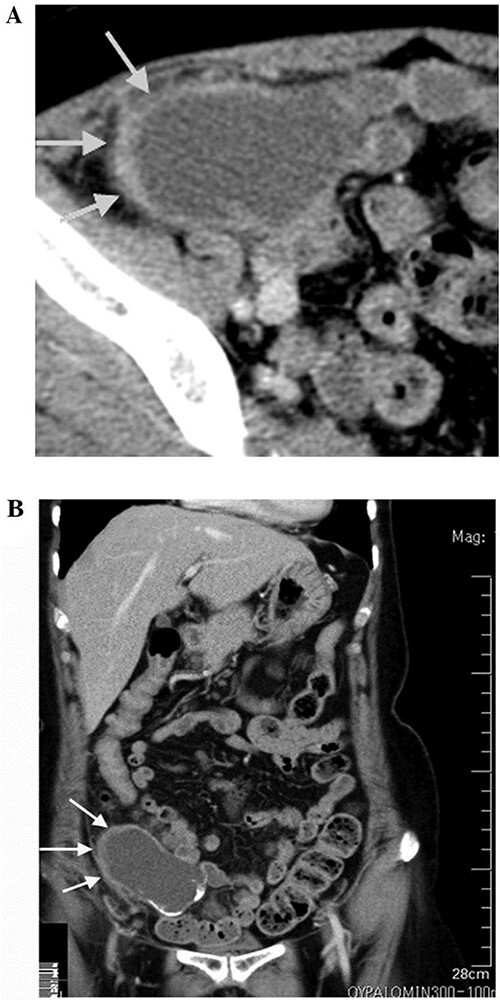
CT scan showing lobulated cystic formation in the right iliac vessels level with an extension to the upper margin of the pelvis. This finding is suspicious, so a decison was made for excision of the tumor and to be sent to pathology department to confirm the diagnosis.
